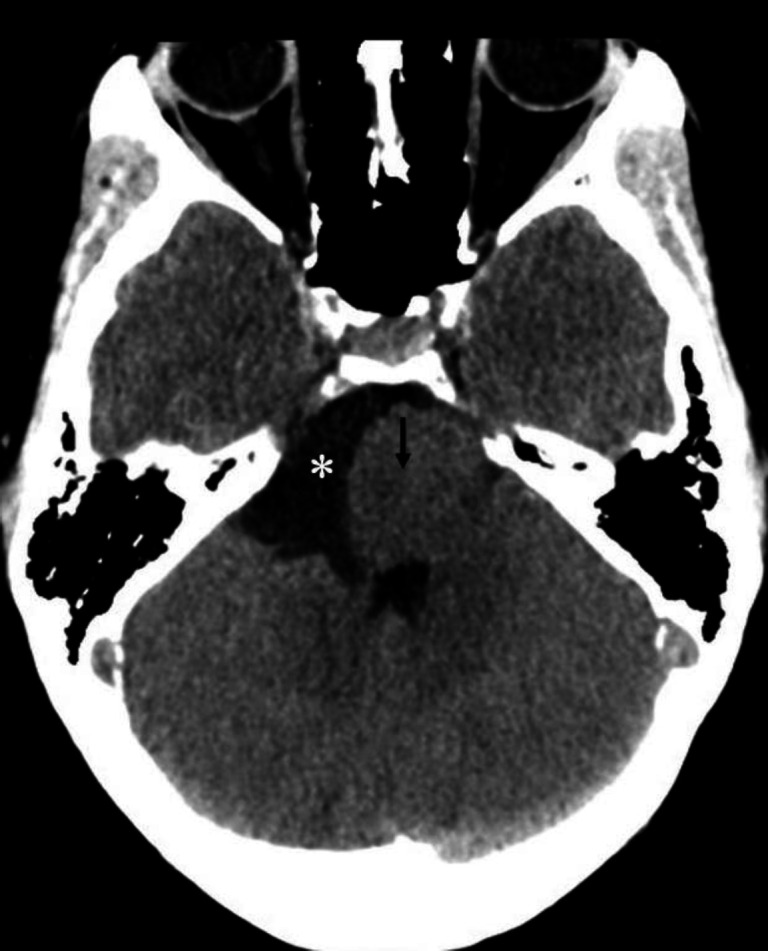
Non-contrast CT brain of a 32 year-old male shows a low density extra-axial lesion (asterisk) in the right cerebellopontine angle region which is compressing the pons (black arrow). Main differential diagnosis of a low density lesion in this location includes an epidermoid cyst and an arachnoid cyst
